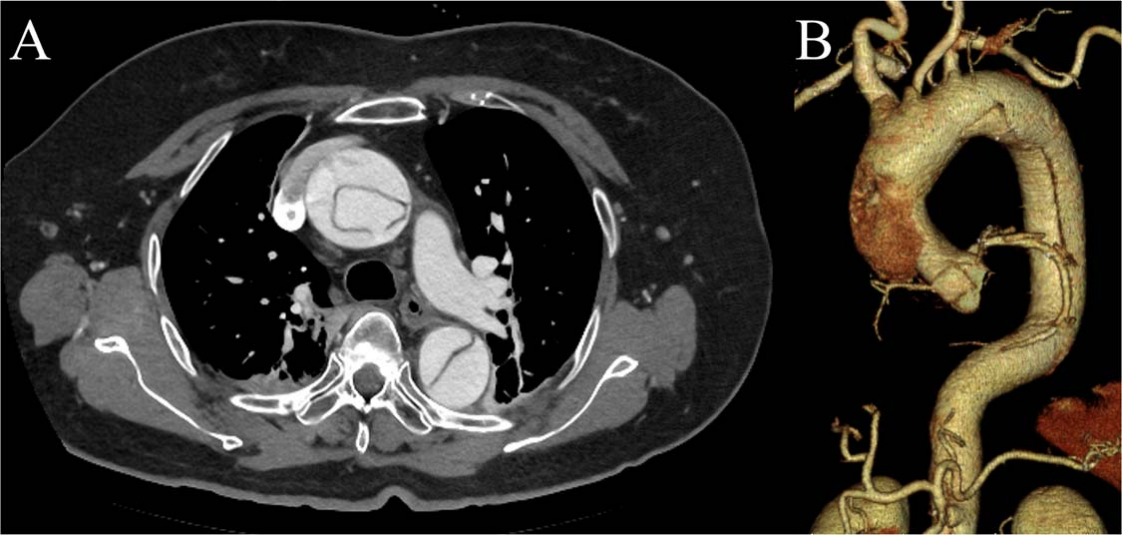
Preoperative contrast-enhanced CT images. (A) Stanford type A acute aortic dissection with the primary entry tear extending from the ascending aorta to the aortic arch, showing a patent false lumen. (B) Three-dimensional reconstructed image.
